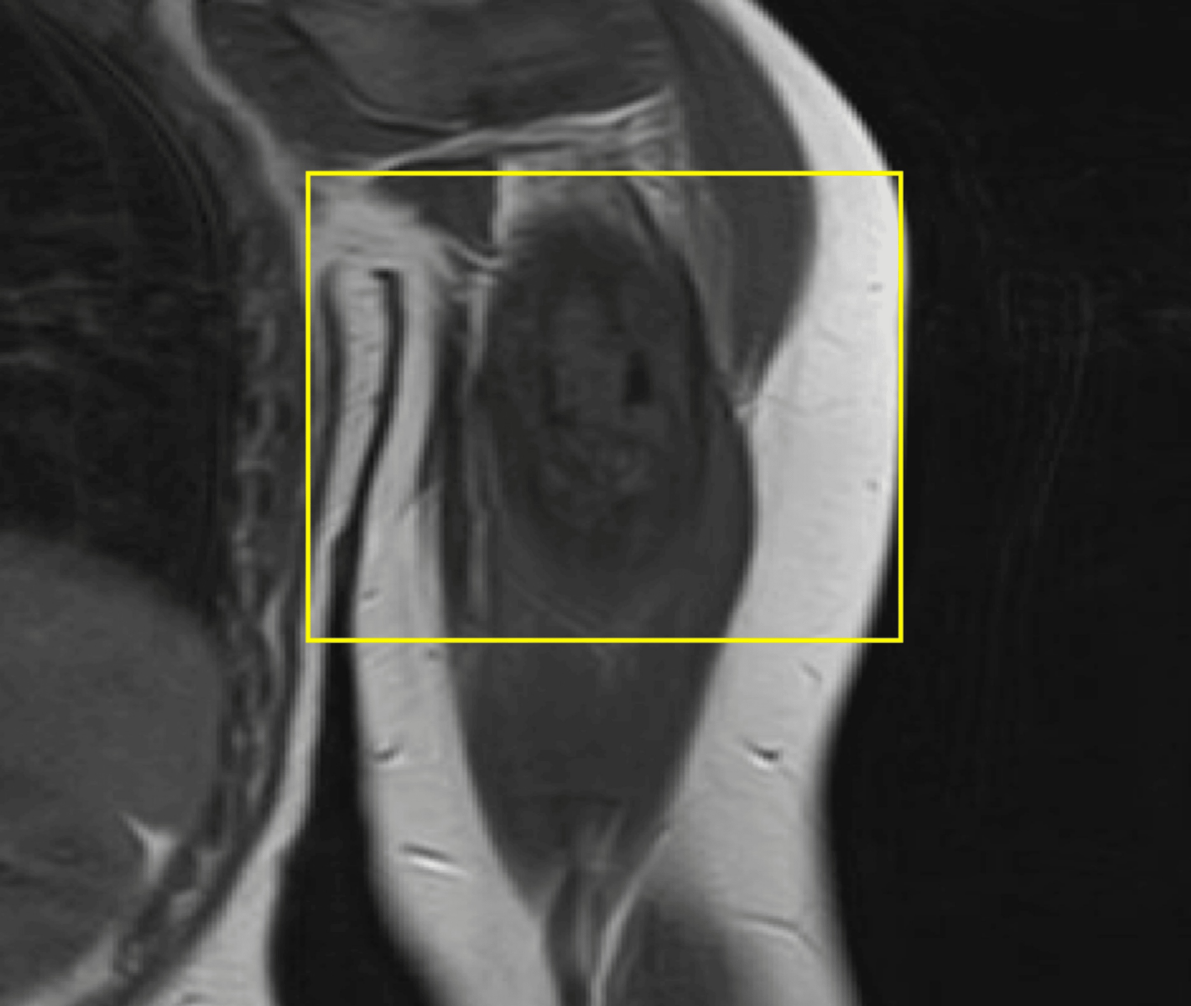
Intraosseous MRI showing enhancing destructive mass arising in the proximal diaphysis of the humerus with significant surrounding periosteal edema, abnormal cortical breakthrough, and periosteal reaction
